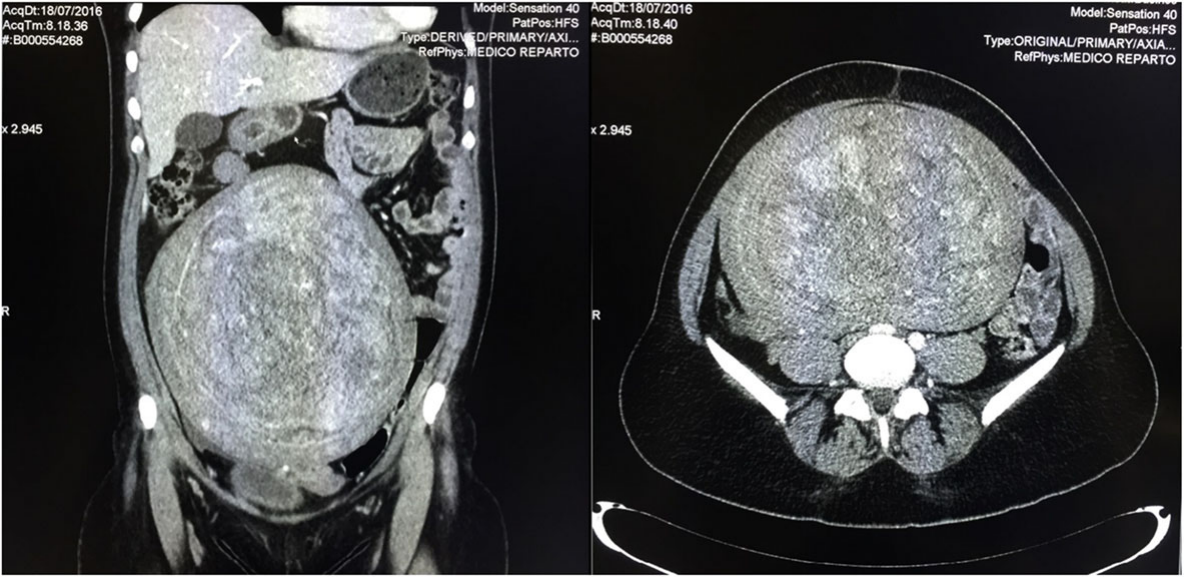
Coronal view and transverse view of computed tomography showing the enlarged uterus occupying the entire abdomen
